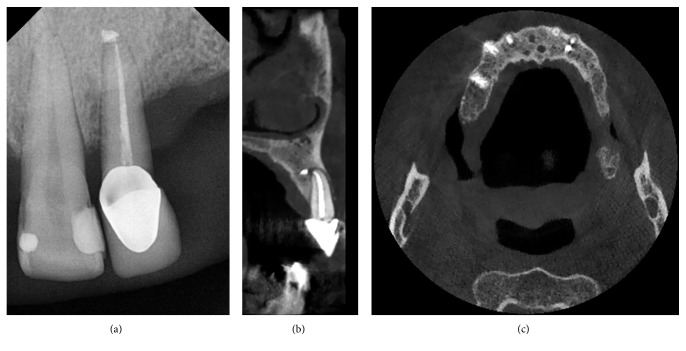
Endodontic treatment 2.2. (a) Periapical X-ray: endodontic overfilling and no periapical radiolucency. (b) CBCT sagittal cross section: apex endodontic treatment, over filling, and periapical radiolucency. (c) CBCT transversal section: over filling and periapical radiolucency.
